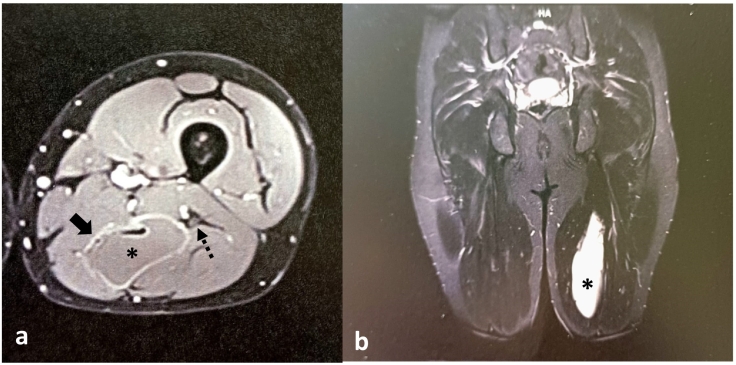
a: Axial cross-sectional image passing through the cyst using T1-weighted imaging with Gadolinium contrast, demonstrating the relationship of the cyst with neural structures. Solid arrow: cyst wall; dashed arrow: sciatic nerve; *: Hydatid Cyst. b: Frontal section in T2-weighted imaging with fat saturation, revealing the hydatid cyst involving the biceps femoris muscle.
